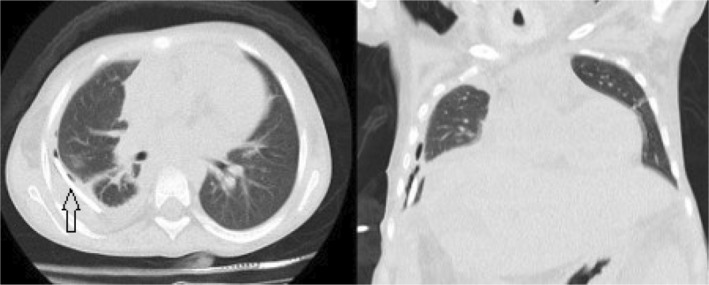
(Left Image) Axial computed tomographic image in soft tissue window demonstrating limited right side pleural effusion 3 days after thoracostomy drain insertion. (Right Image) Coronal computed tomographic image indicating pigtail drain in the right pleural cavity (black arrow).
